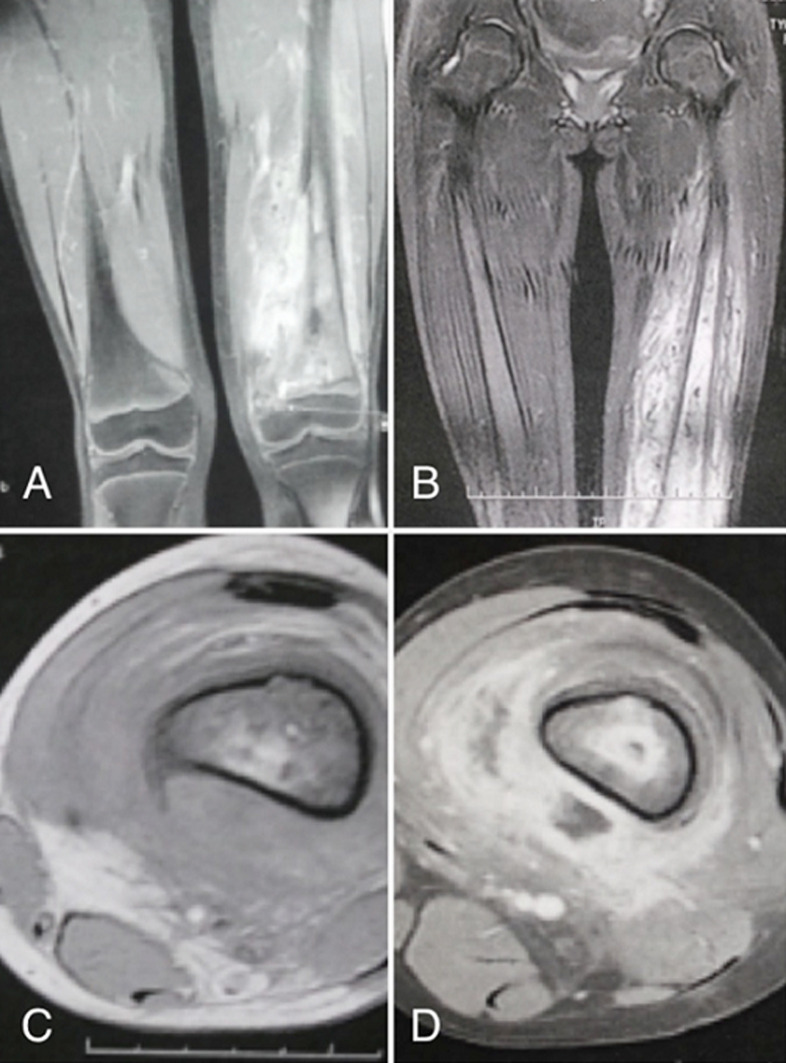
coronal T2 (A), STIR (B) and axial T1 weighted MRI without (C) and with contrast (D) showing metaphyseal-diaphyseal femoral process extending to the soft tissues and to the distal epyphysis
